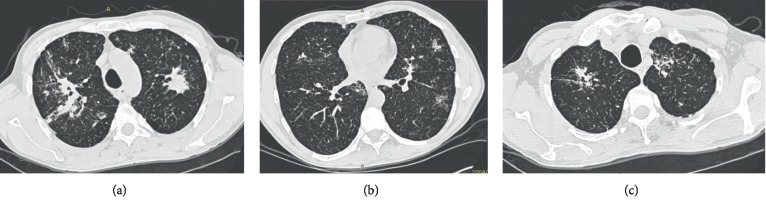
Some examples of lung HRCT with ILD.
